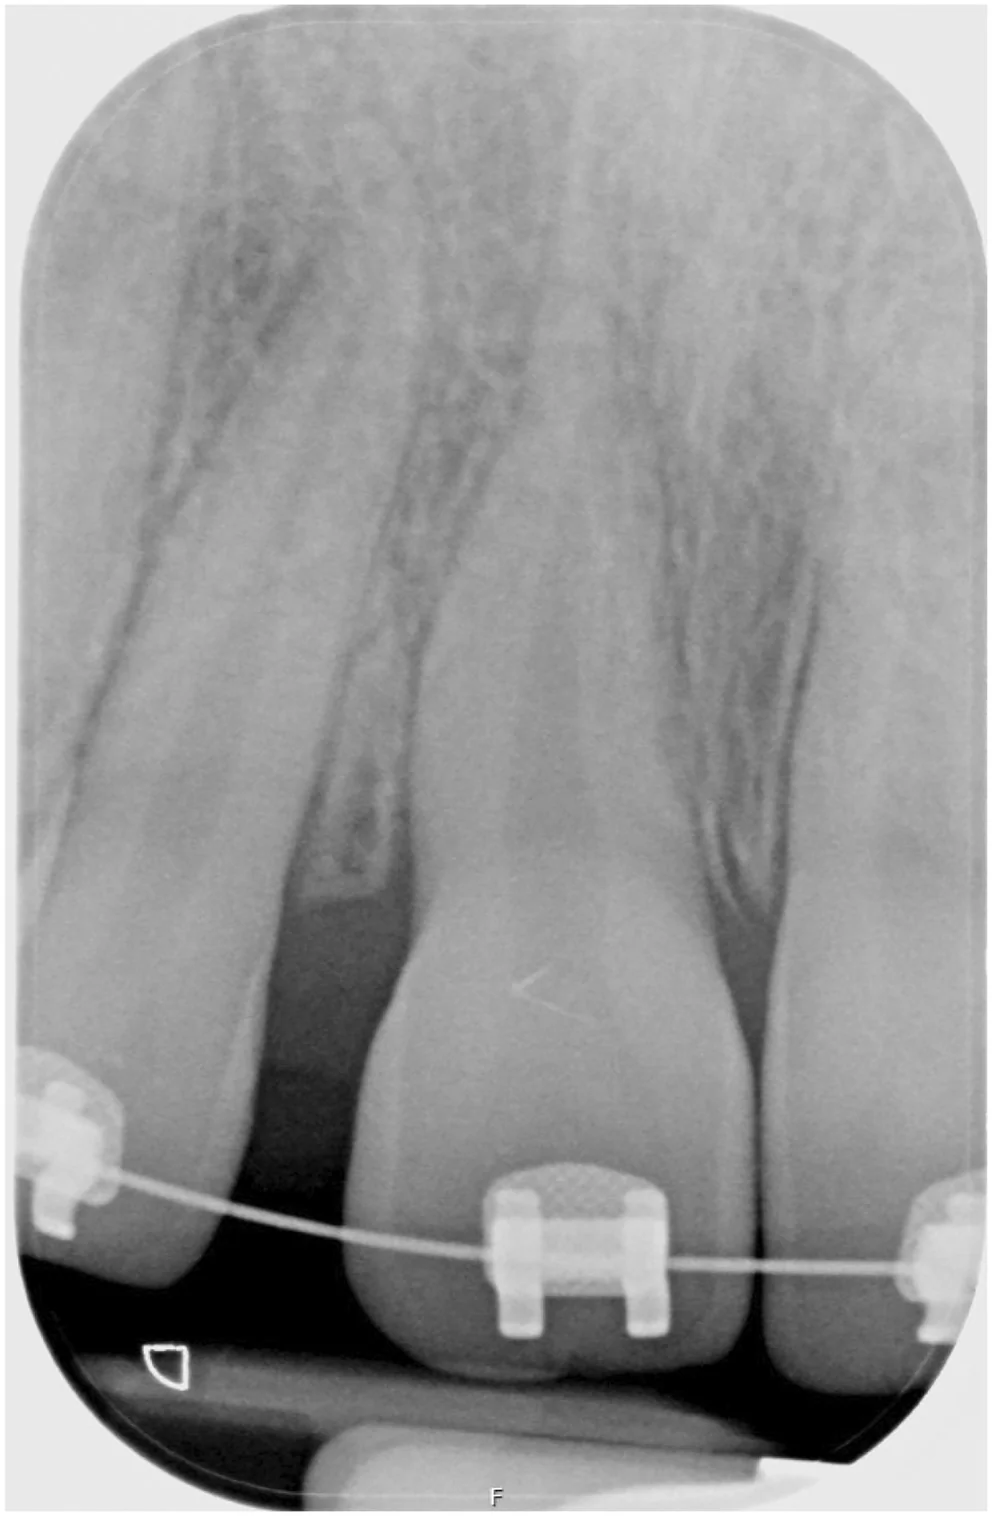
Intra‐oral periapical radiograph of the upper right central incisor taken two months after surgical separation.
